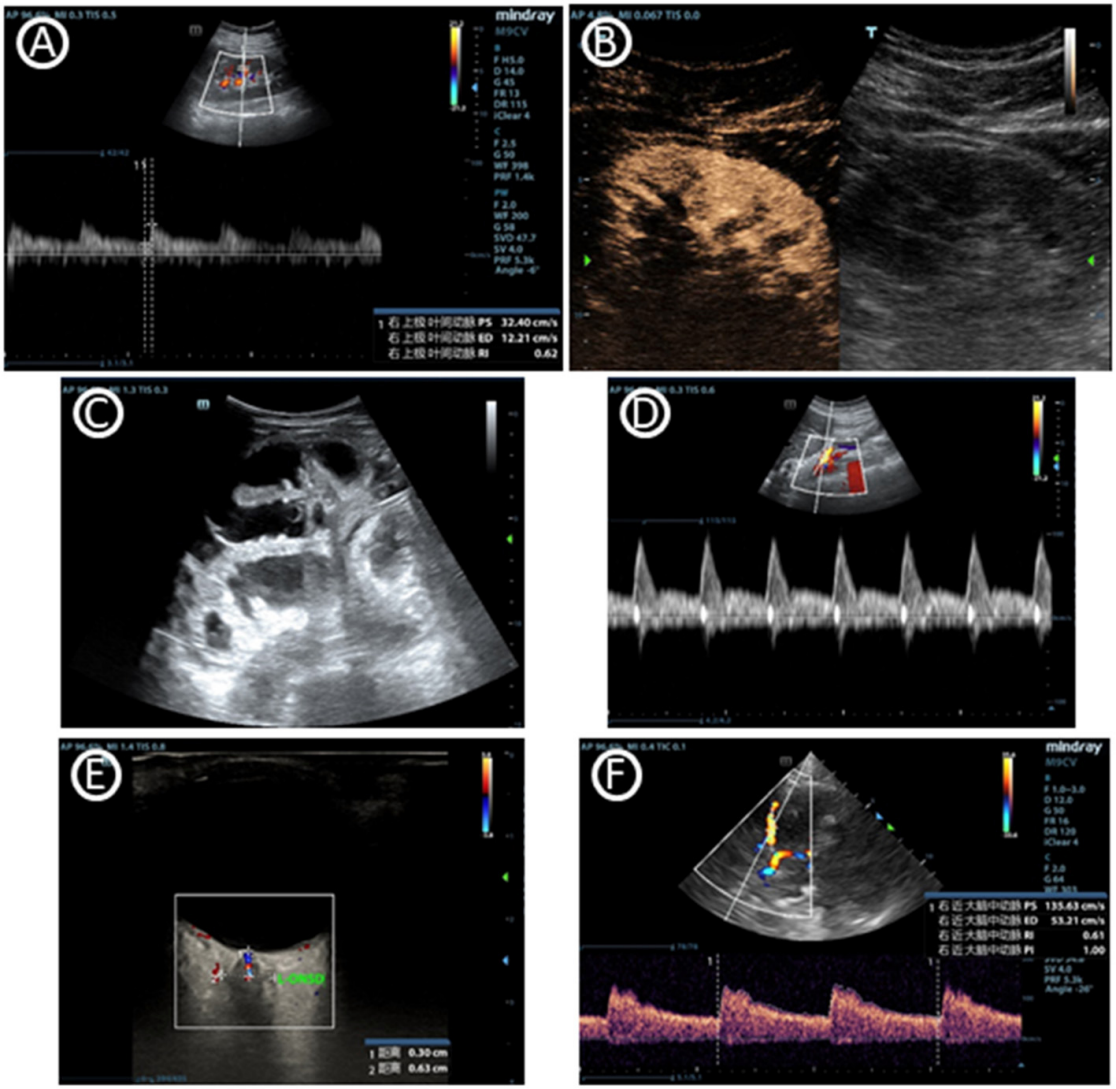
Images of multiorgan ultrasound. (A) Normal doppler tracing of the interlobar vessels in renal ultrasound. The renal resistive index can be measured using the maximal and the minimal arterial velocity during the cardiac cycle. (B) Renal contrast-enhanced ultrasonography. (C) Dilated fluid-filled bowel loops that indicates small bowel obstruction. (D) Blood flow curve of superior mesenteric vein. (E) Optic nerve sheath diameter, which can help to predict intracranial pressure. (F) Cerebral blood flow velocity of middle cerebral artery accessed by transcranial doppler ultrasonography.
